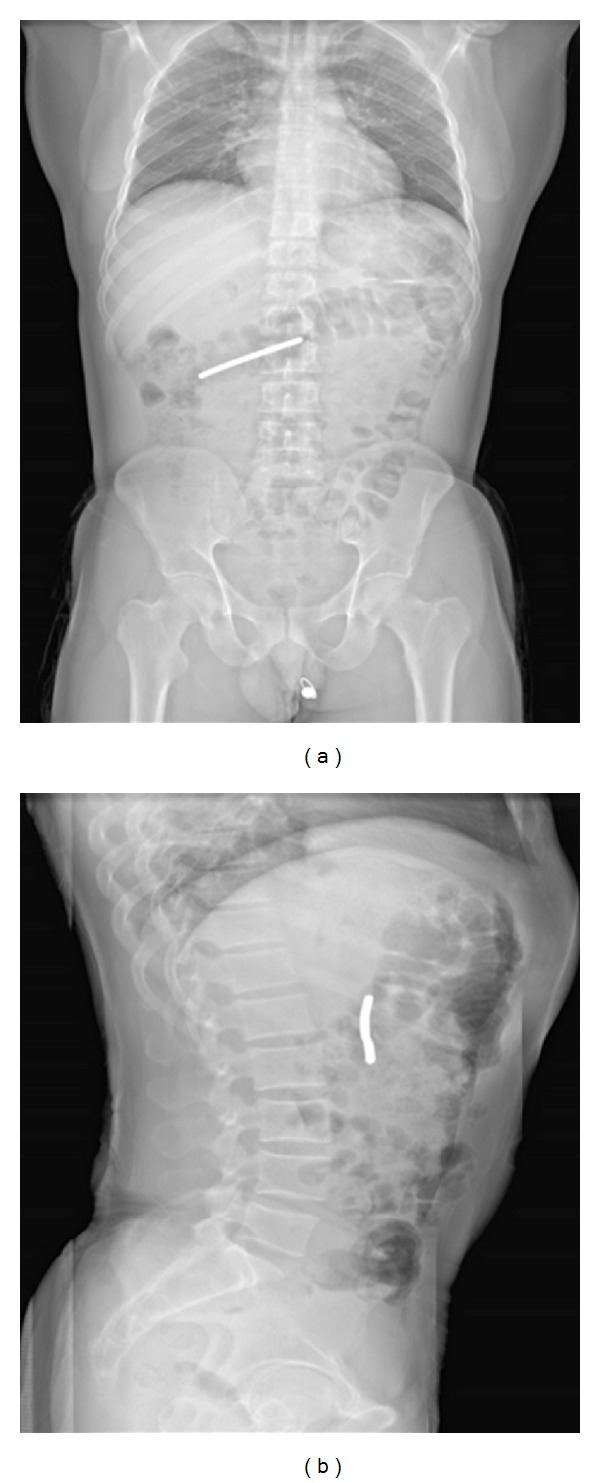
Lodox radiograph showing (a) the AP and (b) the lateral view of an ingested foreign object.
